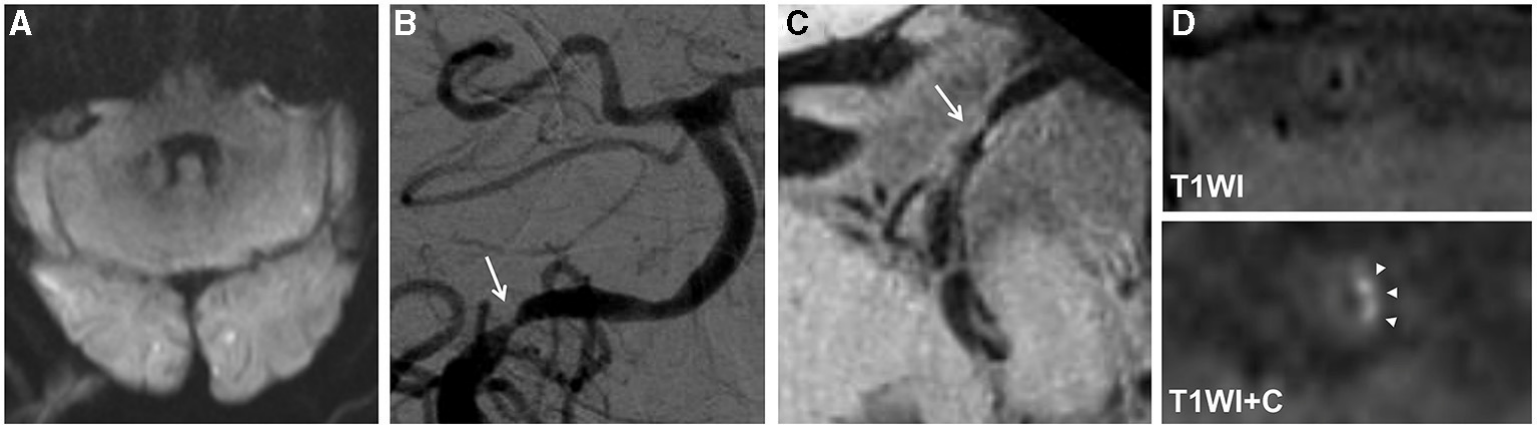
An 80-year-old man presented with dizziness for 15 days. The diffusion-weighted imaging (DWI) showed infarcts of bilateral occipital lobes and left cerebellum (A). The digital subtraction angiography (DSA) showed severe stenosis at the V4 segment of the right vertebral artery (arrow) (B). The high-resolution magnetic resonance imaging (HRMRI) demonstrated a diffuse distributive plaque (arrow) (C) with an eccentric enhancement (arrowheads) on T1-weighted image after gadolinium–DTPA injection (D).
